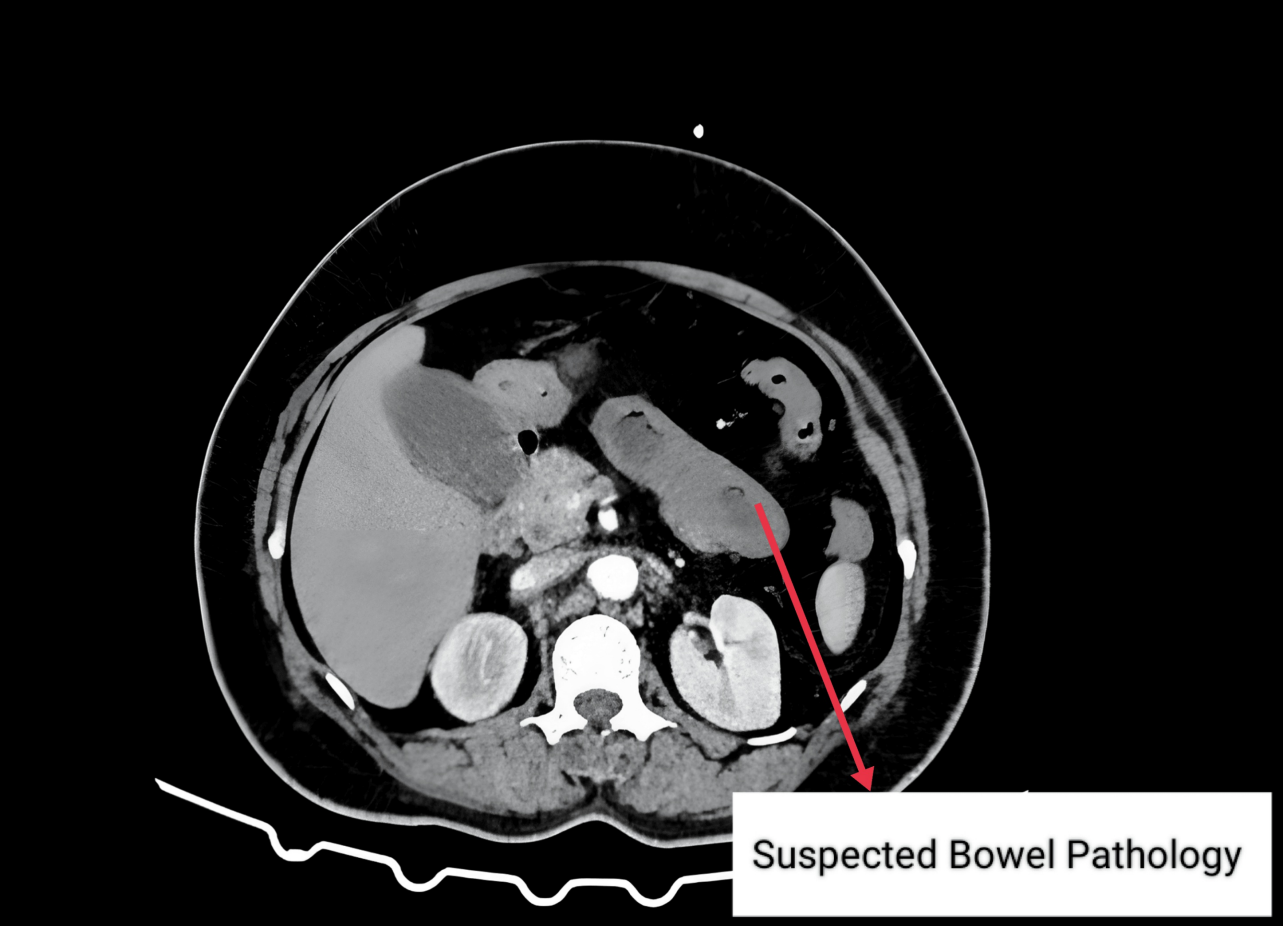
CECT abdomen showing suspected bowel pathology CECT: contrast-enhanced computed tomography
